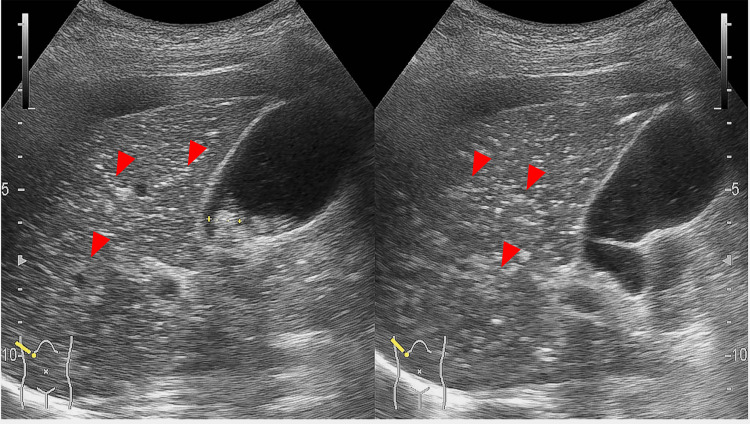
Abdominal ultrasound detection of portal vein gas Abdominal ultrasound image of a 91-year-old woman with non-occlusive mesenteric ischemia. Multiple hyperechoic foci within the liver parenchyma (red arrowheads) are more conspicuous than on computed tomography, consistent with portal vein gas. Numerous hyperechoic foci are distributed throughout both hepatic lobes, extending to within approximately 1 mm of the liver capsule: “fruit-pulp-like” pattern.
